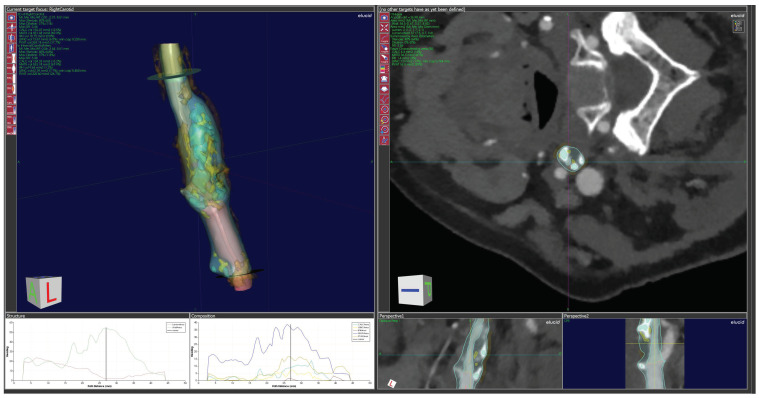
After morphologic segmentation of the lumen and wall in VascuCap and selection of the plaque causing the stenosis, the software-generated 3D model and the longitudinal and cross-sectional image of the lesion are shown in a CTA image.
